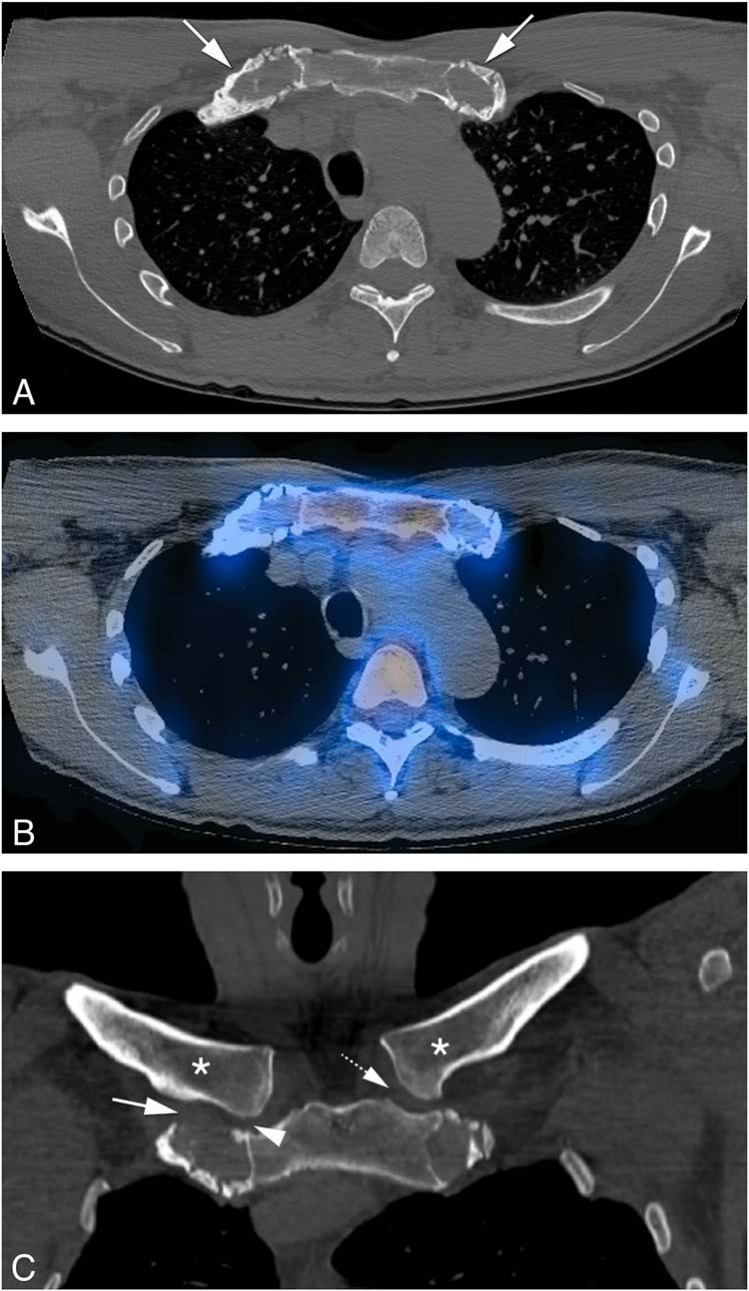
(A) Bilateral hypertrophic ossification of the cartilage and costosternal junctions of the first ribs (arrows). (B) Corresponding SPECT-CT imaging showing no increased uptake in these areas on CT scan, indicating no increase in bone remodeling. (C) Coronal CT imaging showing no abnormalities in the clavicles bilaterally, specifically no sclerosis or hyperostosis (asterisks), no costoclavicular ligament calcification (arrow), and no narrowing of the sterno-clavicular joint space (arrowhead right and dashed arrow left).
